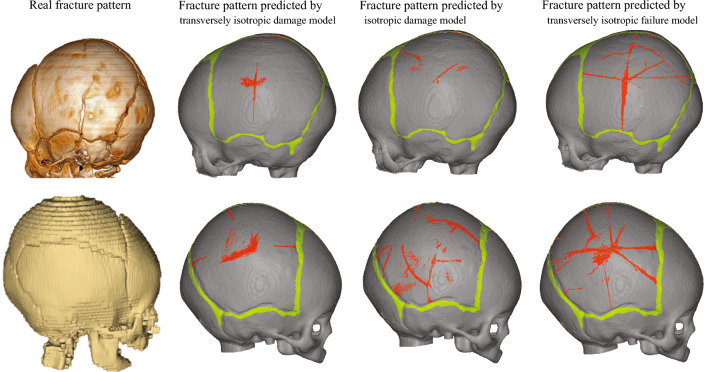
Comparison of fracture patterns in different material models (3 M legal case shown in the upper row, and 4 M legal case in the lower row). In the transversely isotropic material model, material properties along and perpendicular to the fiber direction were respectively fitted based on actual material tests (details in the Sect. 2.2.2). In the isotropic material model, both the material property and damage parameters along the fiber direction were adjusted to match those perpendicular to the fiber direction. Compared with the result by the transversely isotropic material model, the fracture pattern predicted by the isotropic material model differed significantly from the real fracture pattern observed in CT images. In the failure material model, the damage parameters were set to zero to eliminate the ductile properties
